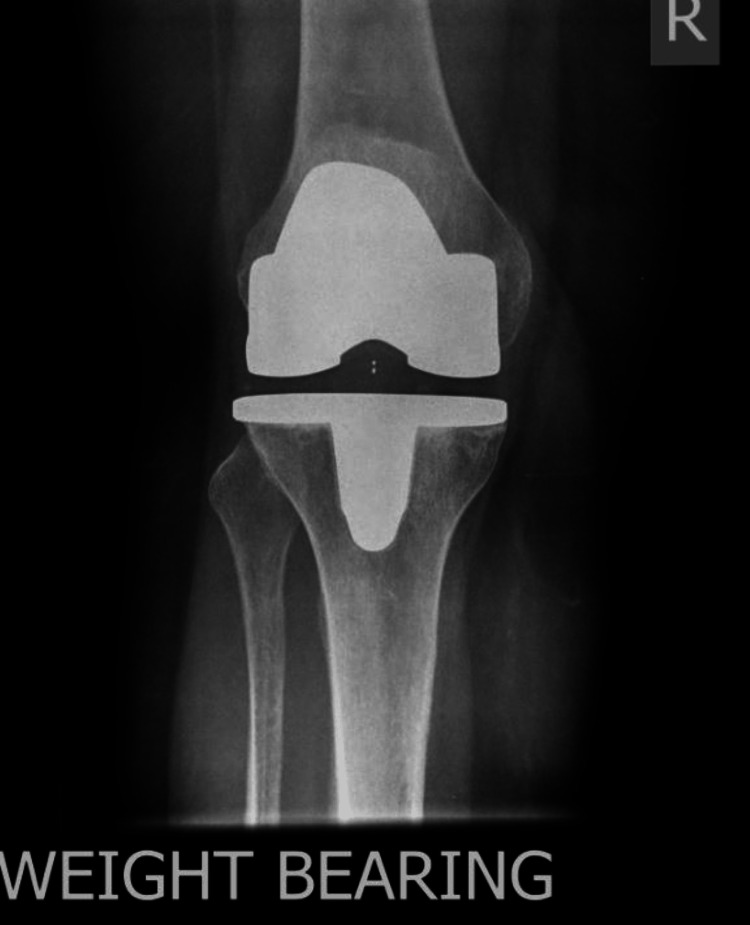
One year postoperative X-ray, AP view AP: anteroposterior
